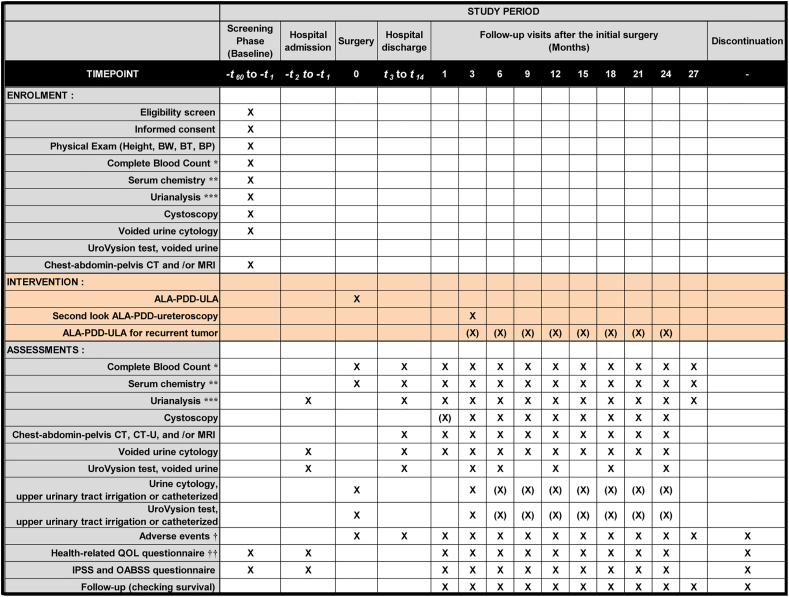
Intervention and assessment schedule of the FLUAM trial. Follow-up visits and data collection should occur approximately 1, 3, 6, 9, 12, 15, 18, 21, 24, and 27 months from the initial surgery. Patients will complete a set of questionnaires at every visit, and follow-up information may be collected via medical charts. The Case Report Form will include information regarding past history, concomitant medications, and any medications taken after the treatment. Chest–abdomen–pelvis computed tomography (CT) and/or magnetic resonance imaging (MRI) should be performed for TNM classification. X, mandatory; (X), optional, ALA-PDD-ULA after 6 months is performed when the radiographic examination suspect tumor recurrence in the upper urinary tract. *hemoglobin, hematocrit, white blood cell count and fractions, platelet count; aspartate transaminase (AST), alanine transaminase (ALT), γ-glutamyl transpeptidase (γ-GTP), total bilirubin, alkaline phosphatase (ALP), lactate dehydrogenase (LDH), total protein, albumin, serum creatinine, blood urea nitrogen, uric acid, total cholesterol, triglyceride, C-reactive protein (CRP), sodium, potassium, chloride; *Urine dipstick test (specific gravity, pH, protein, glucose, bilirubin, urobilinogen, ketone body, and occult blood) and urine sediment test; †according to the Common Toxicity Criteria for Adverse Events (CTCAE v 5.0) translated into Japanese; †† questionnaires SF-8™, EORTC QLQ-C30, and FACT-BL. Abbreviations: BW, body weight; BT, body temperature; BP, blood pressure; ALA, 5-aminolevulinic acid; PDD, photodynamic diagnosis; ULA, ureteroscopy laser ablation; QOL, quality of life; IPSS, International Prostate Symptom Score; OABSS, overactive bladder symptom score.
